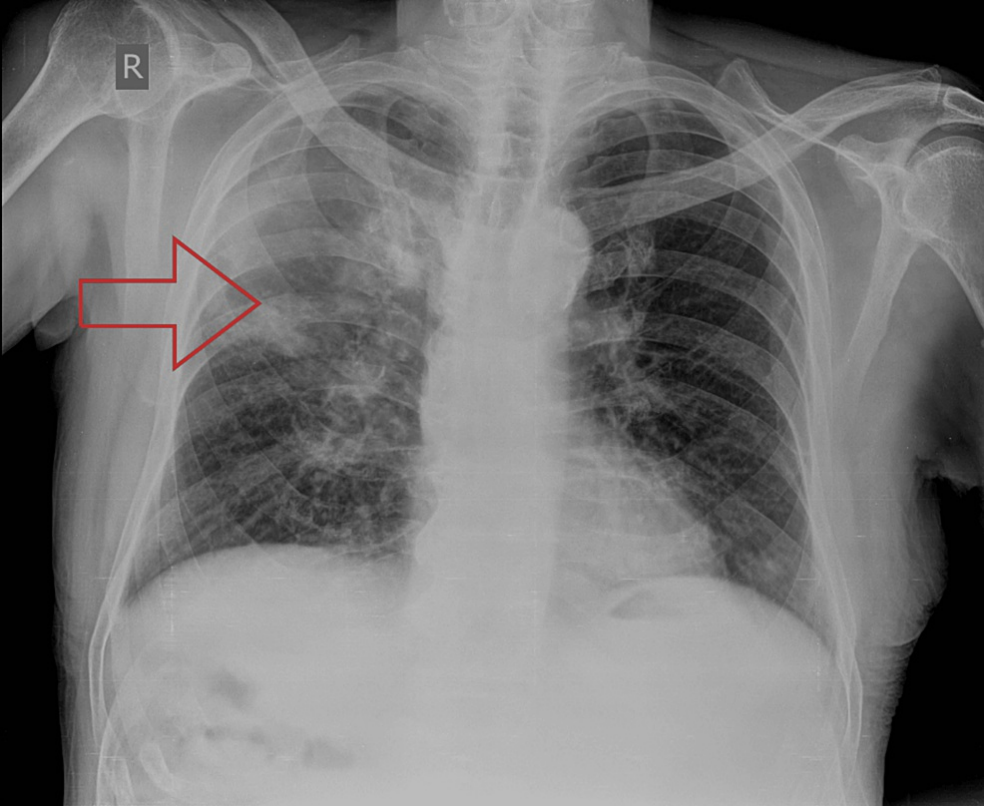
Chest X-ray PA view showing inhomogeneous opacities in the right upper lobe (red arrow) PA: posteroanterior
